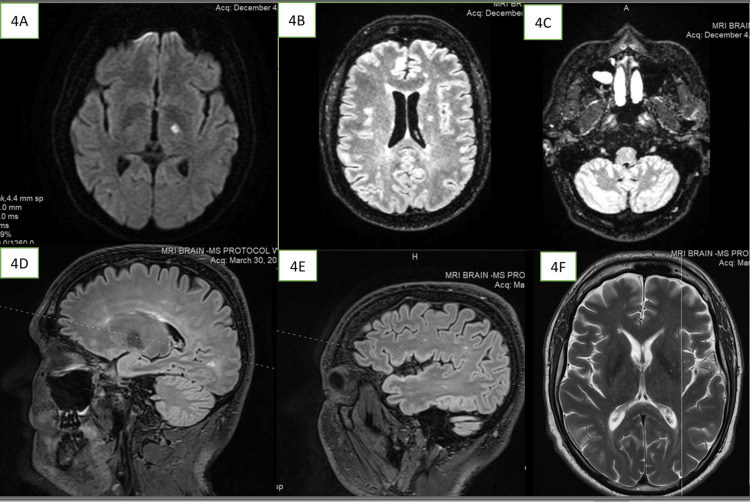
Brain MRI in December 2022 showing newly appearing lesions (4A) An obvious FLAIR hyperintense lesion in the left anterior thalamus. (4B) Multiple lesions in the periventricular and deep white matter showing diffusion restriction. (4C) One lesion in each of the cerebellar hemispheres showing diffusion restriction. (4D, 4E, and 4F) Multiple small lesions in the subcortical and deep white matter FLAIR: fluid-attenuated inversion recovery
